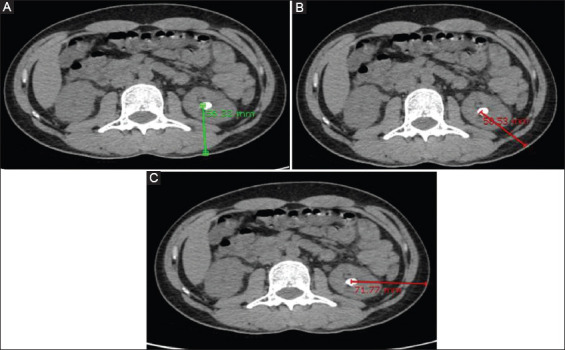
(A) The computation of skin-to-stone distance at 90° on an axial scan of non-contrast computed tomography. SSD: Skin-to-stone distance. (B): The computation of skin-to-stone distance at 45° on an axial scan of non-contrast computed tomography. SSD: Skin to stone distance. (C): The computation of skin-to-stone distance at 0° on an axial scan of non-contrast computed tomography. SSD: Skin-to-stone distance. Average of skin to stone distance measured at three angles (0°, 45, and 90°) was considered as the skin to stone distance.
